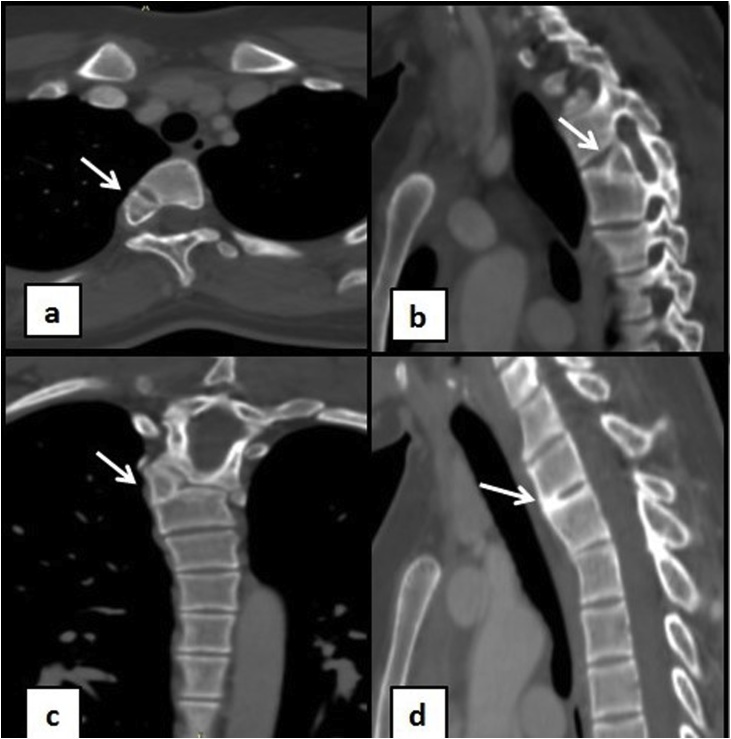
Different CT chest views depicting a 4th hemivertebra. 3a: Axial image, 3b: Sagittal image, 3c: Coronal image showing subtle scoliosis, 3d: Sagittal CT chest image shows a partial block T2 and T3 vertebrae.
